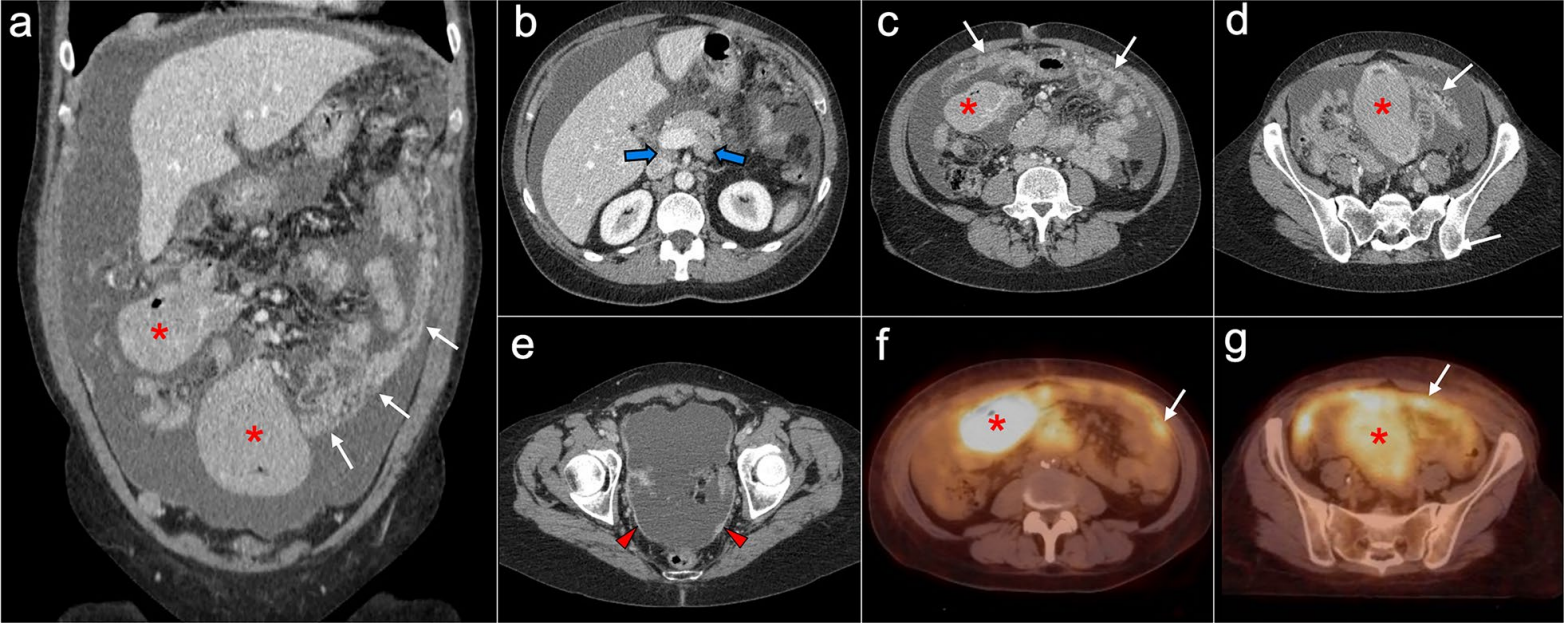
Peritoneal lymphomatosis in a 52-year-old woman, who had been complaining of abdominal distension and nausea for 3 months. Coronal (a) and axial (b–e) CT images in the portal phase show ascites, confluent nodularity of the omental fat with omental cake appearance (white arrows), thickening of the peritoneal folds (red arrowheads), retroperitoneal lymphadenopathies (blue arrows) and two bulky mesenteric masses that produce encasement of the mesenteric vessels and the adjacent small bowel loops (red asterisks). f, g Fusion PET-CT images show significant standard uptake value of the bulky masses (red asterisks) and the omental cake (white arrows). The final diagnosis of follicular non-Hodgkin's lymphoma was made by surgical biopsy of the mesenteric masses, which corresponded to adenopathic conglomerates
